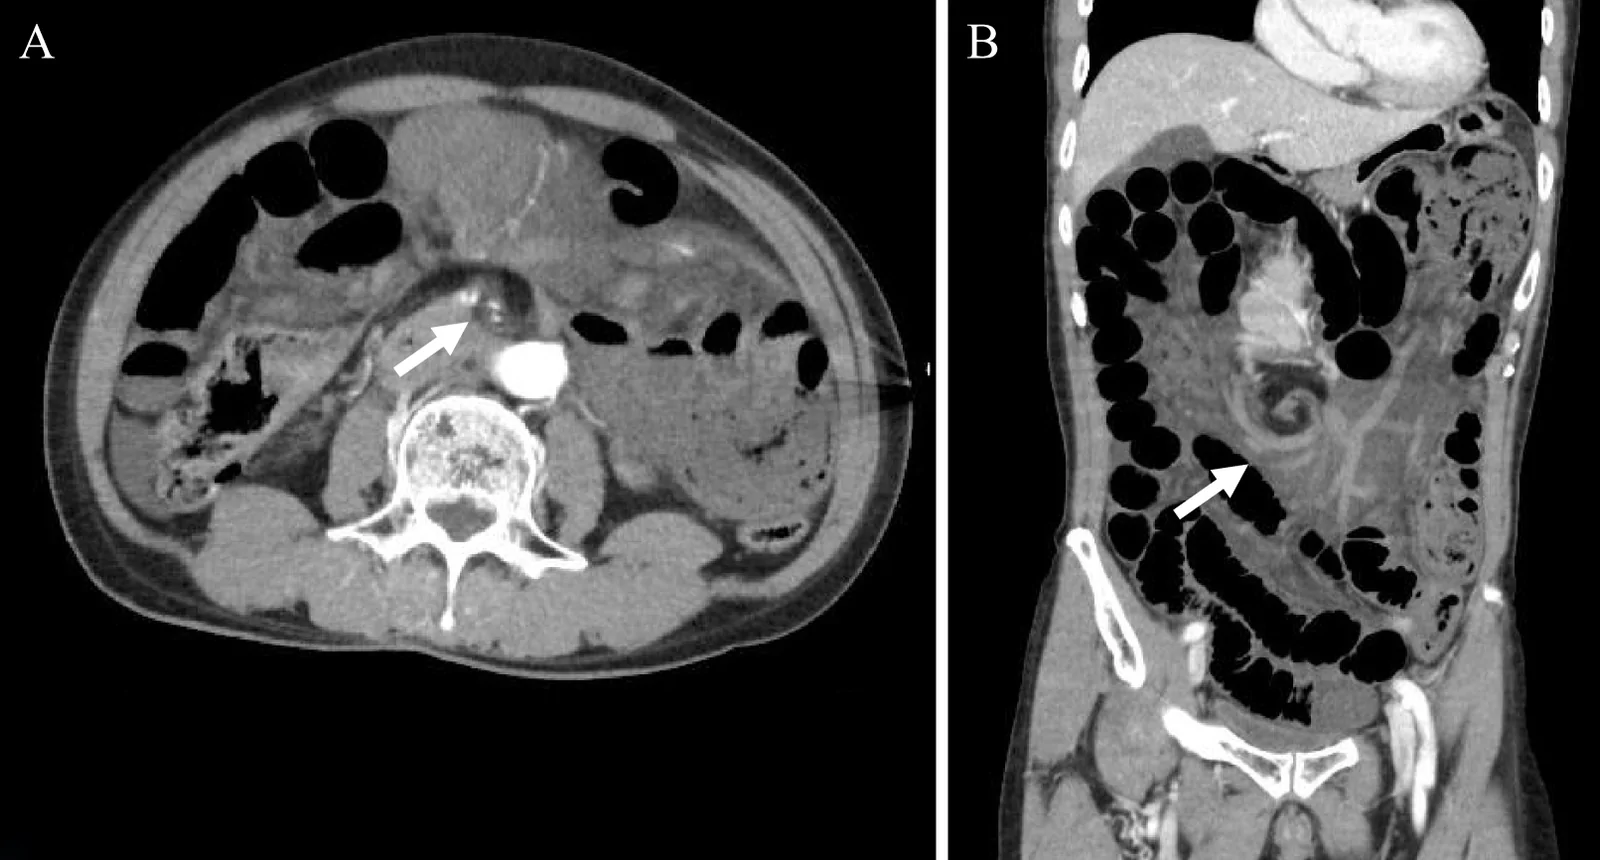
Contrast-enhanced computed tomography. (A) Axial plane; (B) coronal plane. The white arrows indicate the whirl sign in the mesentery, consistent with small bowel volvulus.
